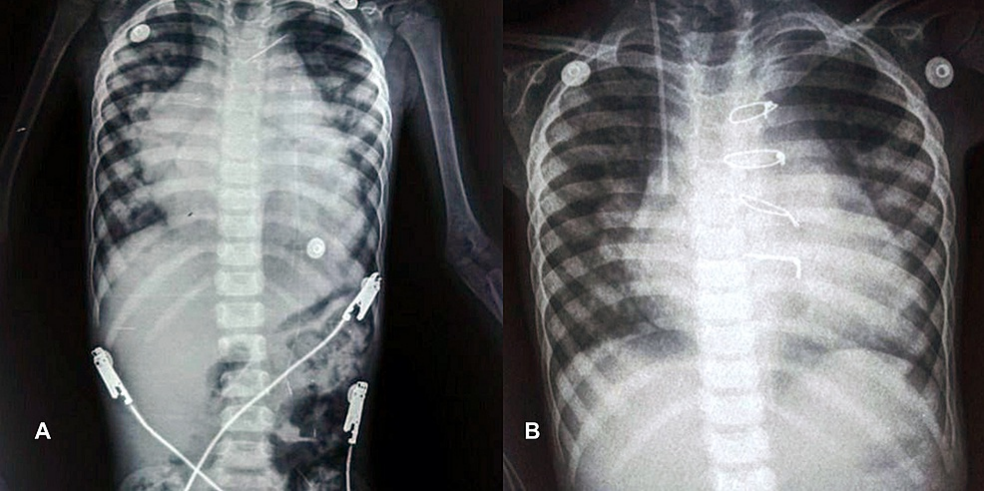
Preoperative (A) and postoperative (B) chest x-ray of the three-year-old girl
